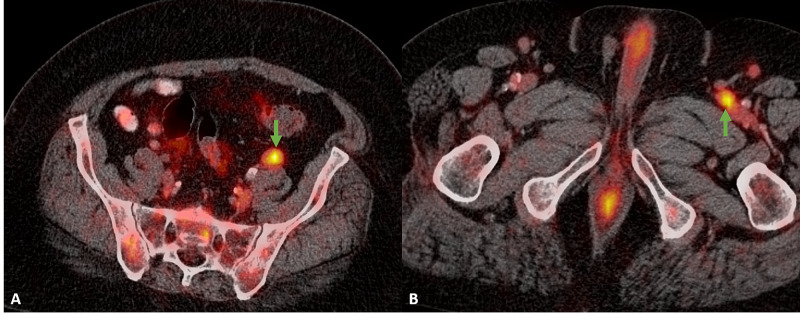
Pelvic PET/CT Images (A) and (B) demonstrate bilateral hypermetabolic inguinal lymph nodes (green arrows). PET/CT, Positron emission tomography/computed tomography.
